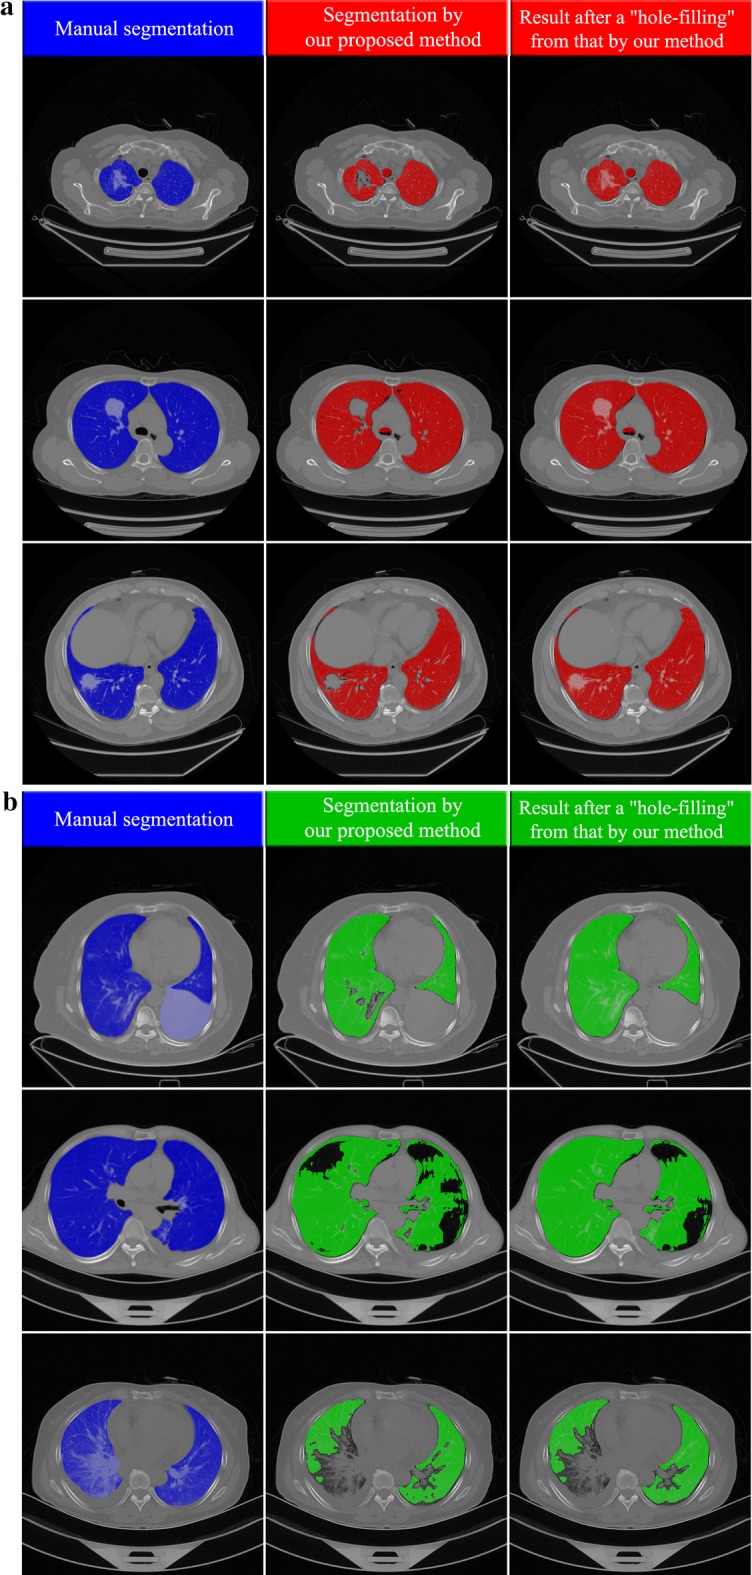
Examples of segmentation at axial slices (In each sub-figure, there are three columns: the left the left column shows the results of manual segmentation of lung field including both lung parenchyma and lesions; the middle column presents the results of segmentation of lung parenchyma by our proposed method; the right column shows the results after a “hole-filling” operation from those by our method.). a Three example slices (three rows) for subjects with lung cancer (PET-CT scanner). b Three example slices (three rows) for special cases where the pleural effusion, emphysema, inflammation are near the boundary the lung field
